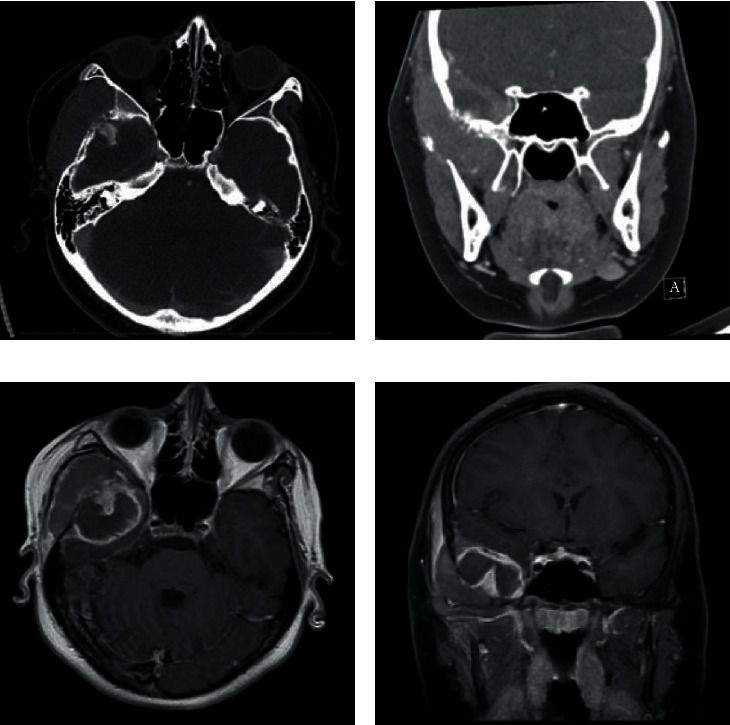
Lytic changes and destruction of sphenoid bone with adjacent right middle cranial fossa mass. (a) Head CT: bone window focusing on the destruction of the right sphenoid wing. (b) Head CT: brain window focusing on 3.0 × 4.4 × 2.4 cm peripherally enhancing mass in the right middle cranial fossa. (c, d) Brain MRI showing 2 × 2.9 × 5.2 cm right temporal region mass and irregularity under the right sphenoid wing and an adjacent 4.5 × 3.1 × 2.5 cm intracranial mass.
